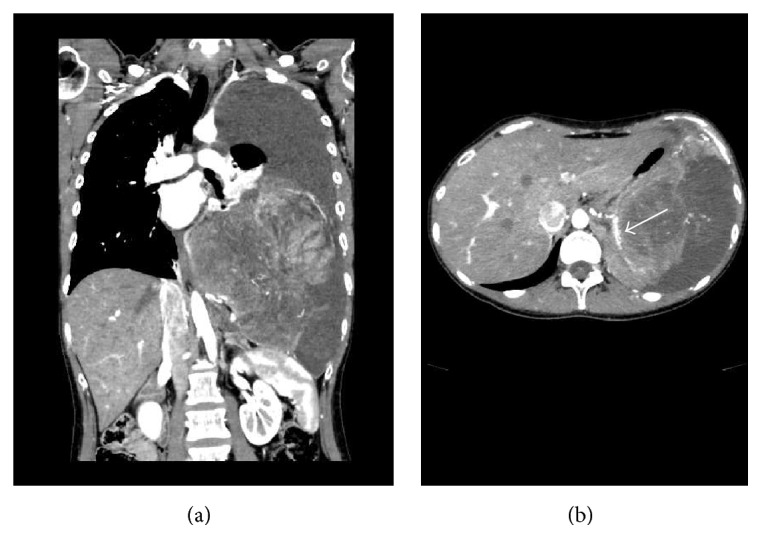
(a) Chest contrast-enhanced computed tomography (CT) showing the bulky intrathoracic mass opacity on the left. (b) The inferior phrenic artery was found to be the main nutrient vessel in the contrast-enhanced CT (arrow).
